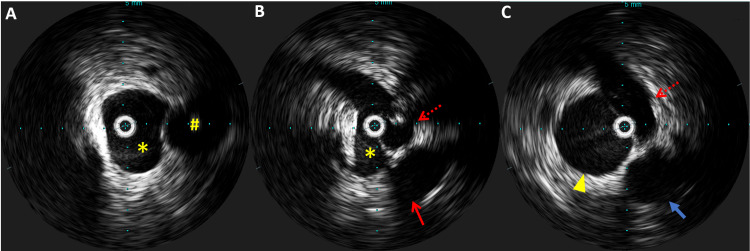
Intravascular ultrasound of left common iliac vein Intravascular ultrasound of iliac vein before venous stent placement. (A) Left common iliac vein (asterisk) and left common iliac artery (hashtag) at the proximal level to the compression; (B) left common iliac vein (asterisk) compressed by the left internal iliac artery (dotted arrow) at the level of external compression; (C) left external iliac vein (arrowhead) at the level of post obstruction and the thin head arrow showing left external iliac artery.
